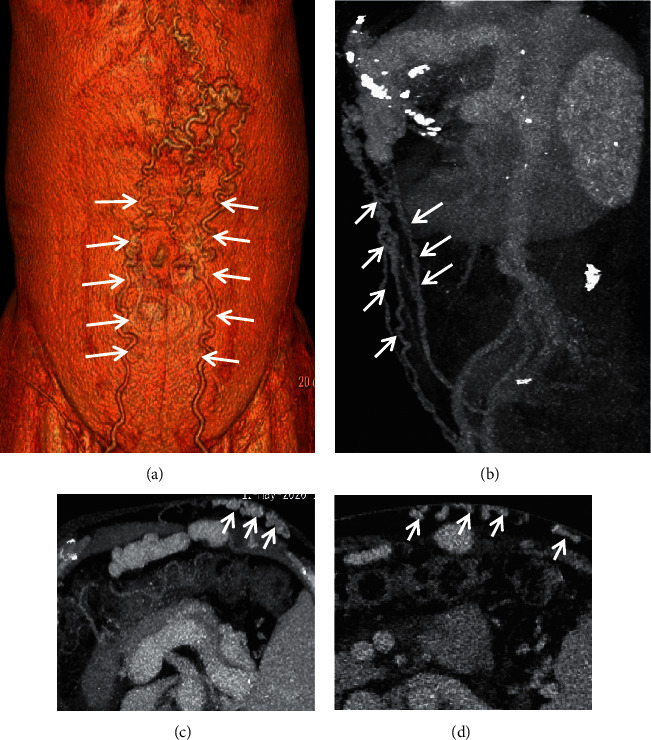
Abdominal wall varices. In a 65-year-old female with liver cirrhosis, the contrast-enhanced CT scan on the portal vein phase demonstrated that abdominal wall varices (white arrow) manifested as dilated, enhanced, and tortuous structures. (a) Three-dimensional reconstruction; (b) sagittal contrast-enhanced CT; (c, d) axial contrast-enhanced CT.
